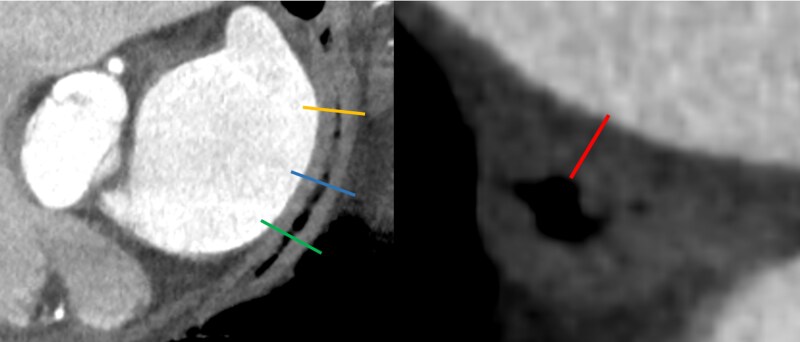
Representative computed tomography image showing the assessment of the anatomical parameters of the left atrial posterior wall and the oesophagus. Left: venous phase angiography shown in the sagittal plane through the oesophagus. Yellow, blue, and green lines show the levels of superior, mid-, and inferior measurement levels. Right: axial plane image showing the distance between the left atrial posterior wall endocardium and the oesophageal mucosa at the point of measurement (corresponding to the level of the blue line on the left figure).
